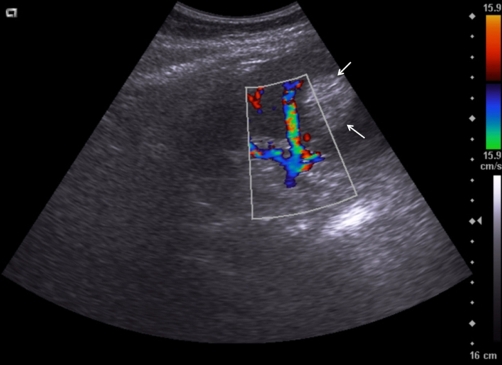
Abdominal ultrasound a month after surgery showing good vascularisation and incorporation of the omentum (between arrows) to the splenic tissue.
